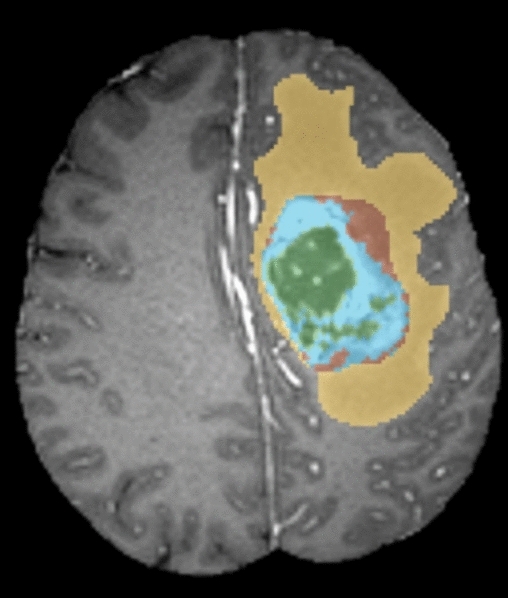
Typical tumor-related MR image.
